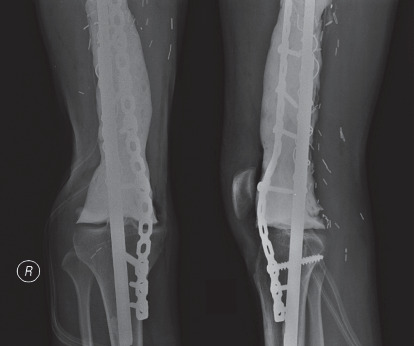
Postoperative radiographs of a 23-year-old male patient (case number 4) who underwent intra-articular resection and knee arthrodesis using the metallic-plus-bone-cement method.
